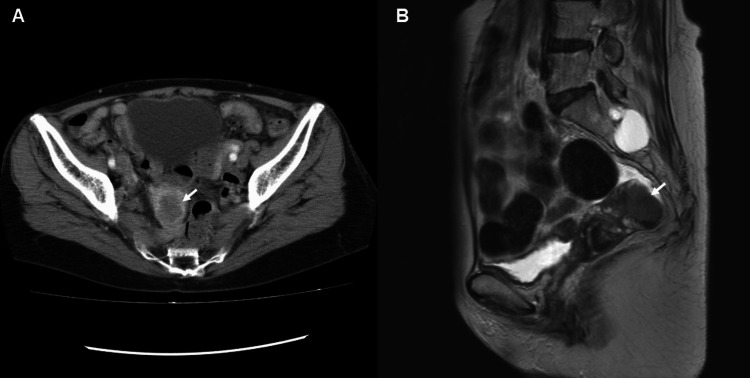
The images one year after the initial operation. (A) A CT image showing a myometrial nodule measuring 3 cm in diameter (arrow).
